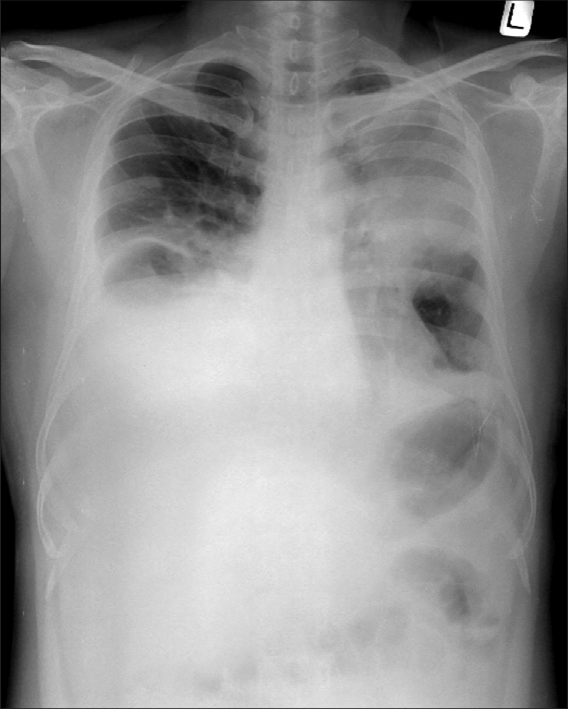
Chest radiograph showing increase in the size of left upper lobe mass with underlying collapse and increased pleural effusion on right side as compared to the previous chest radiograph
